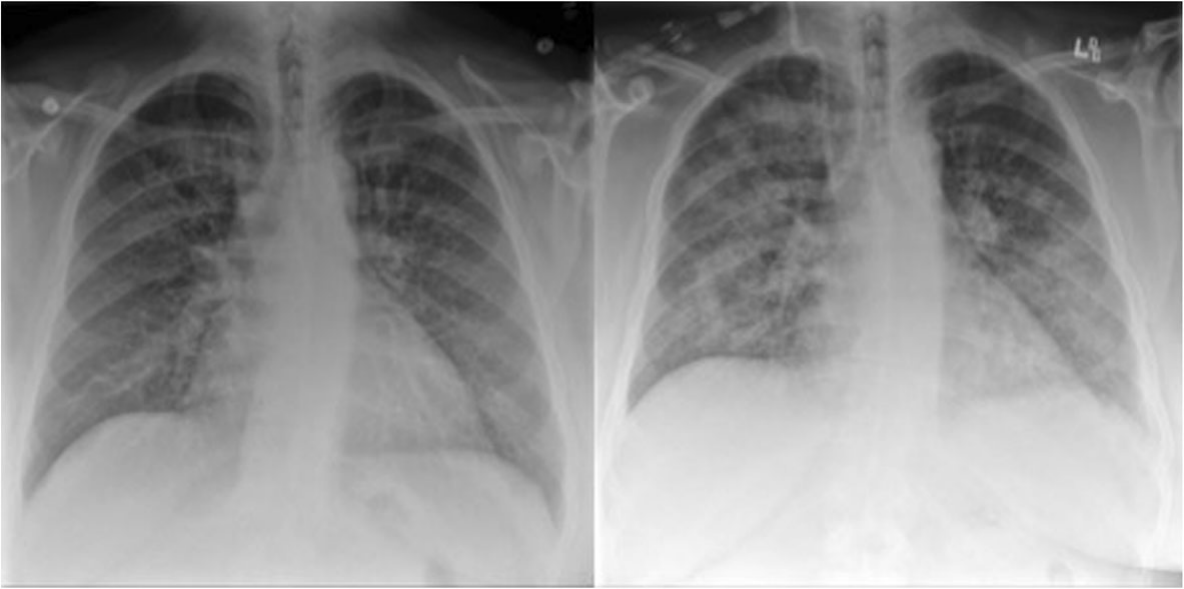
Left image shows the chest radiograph of the patient at initial presentation. Right image shows worsening of bilateral diffuse opacities at second presentation, found later to be related to CMV pneumonitis. Patient’s chest radiograph improved after initial presentation although not shown
